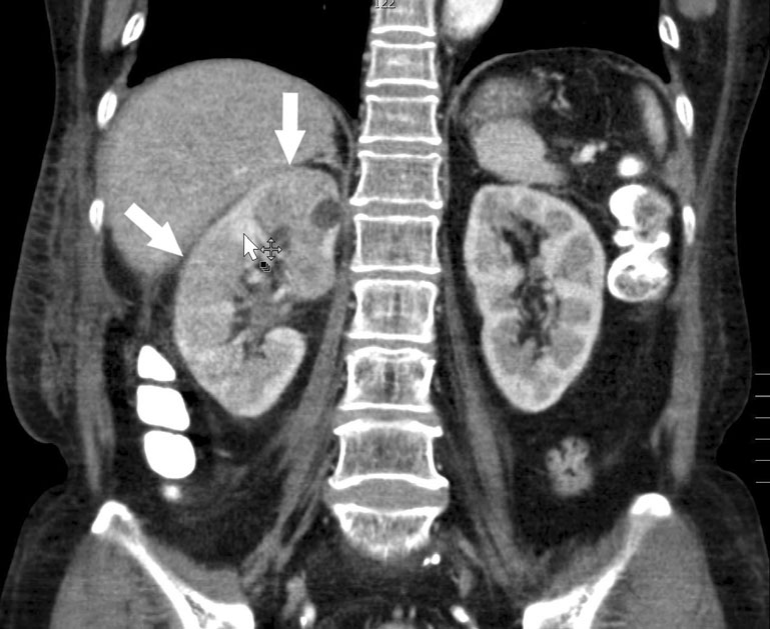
Sixty-five-year-old female with multiple myeloma being treated with a combination chemotherapy regimen that included carmustine. Post-chemotherapy CT showing bilateral enlarged kidneys and a hypo-attenuating lesion in the right kidney (*arrows*) consistent with interstitial nephritis
